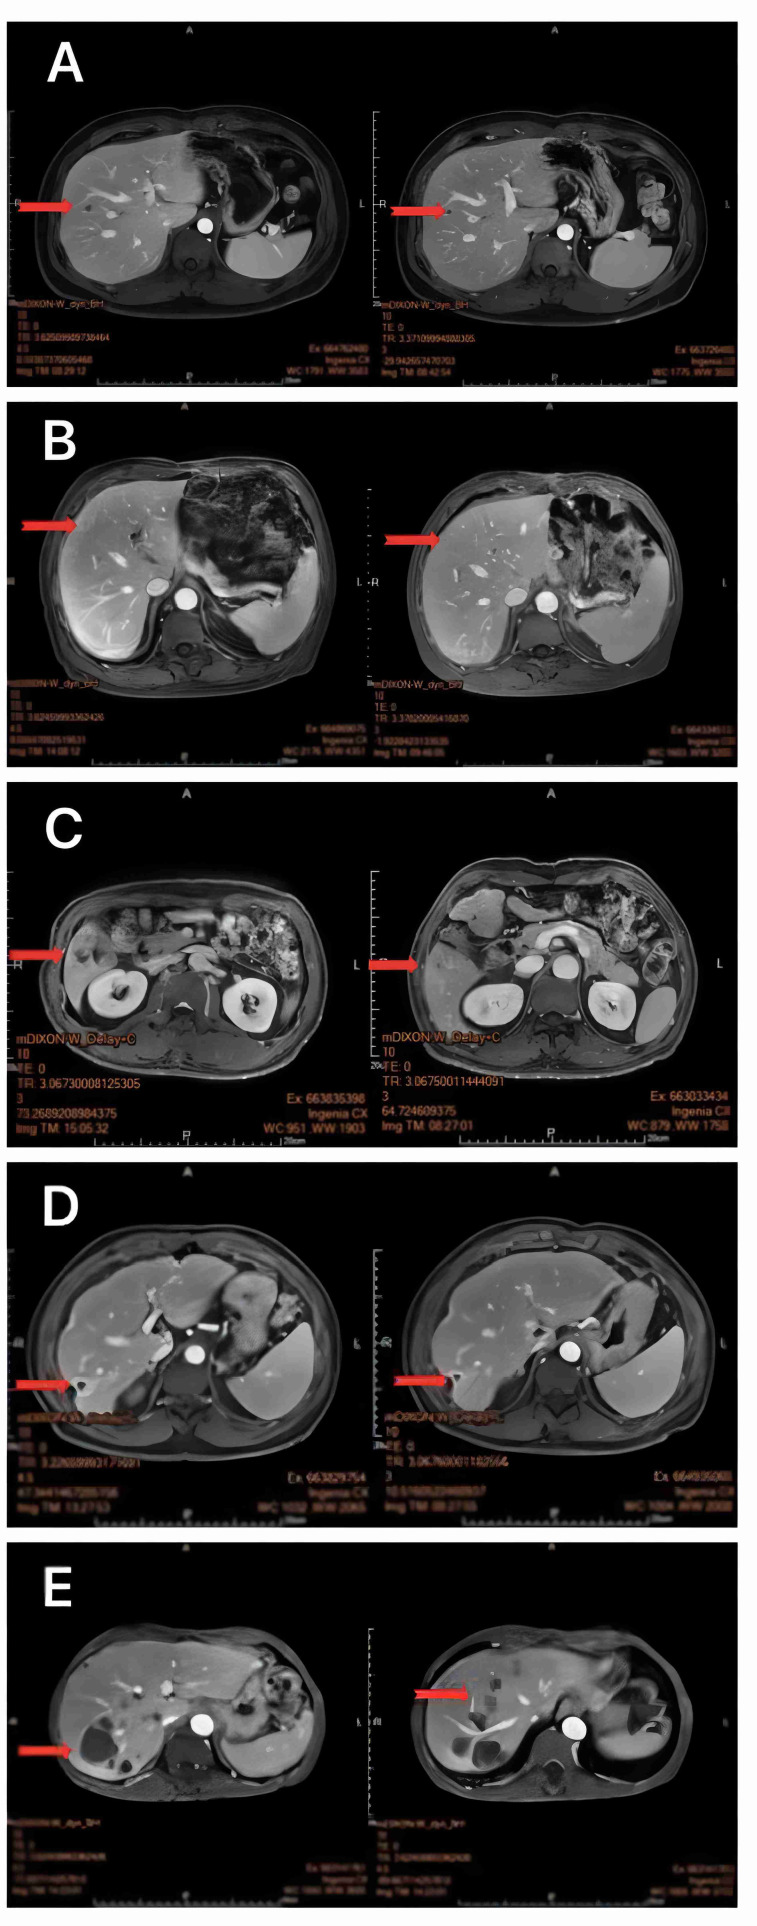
MRI of the liver 1 month after oncolytic virus injection showed a marked reduction in target organ shading (A–E).
